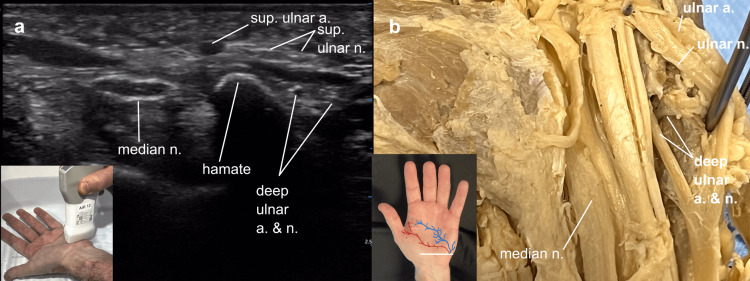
Deep ulnar branches Deep ulnar branches: (a) Ultrasound view of a left hand over the ulnar (Guyon’s) canal with the probe marker directed towards the left (thumb) for better correlation with the cadaver view, demonstrating the deep ulnar artery and nerve traveling beneath the hypoechoic hypothenar muscles, (b) cadaver demonstration of the deep ulnar artery and nerve piercing the proximal hypothenar muscles, hand inset image: blue line representing deep ulnar nerve innervating interossei, adductor, and hypothenar muscles, red line representing deep ulnar artery communicating with deep palmar arterial arch.
